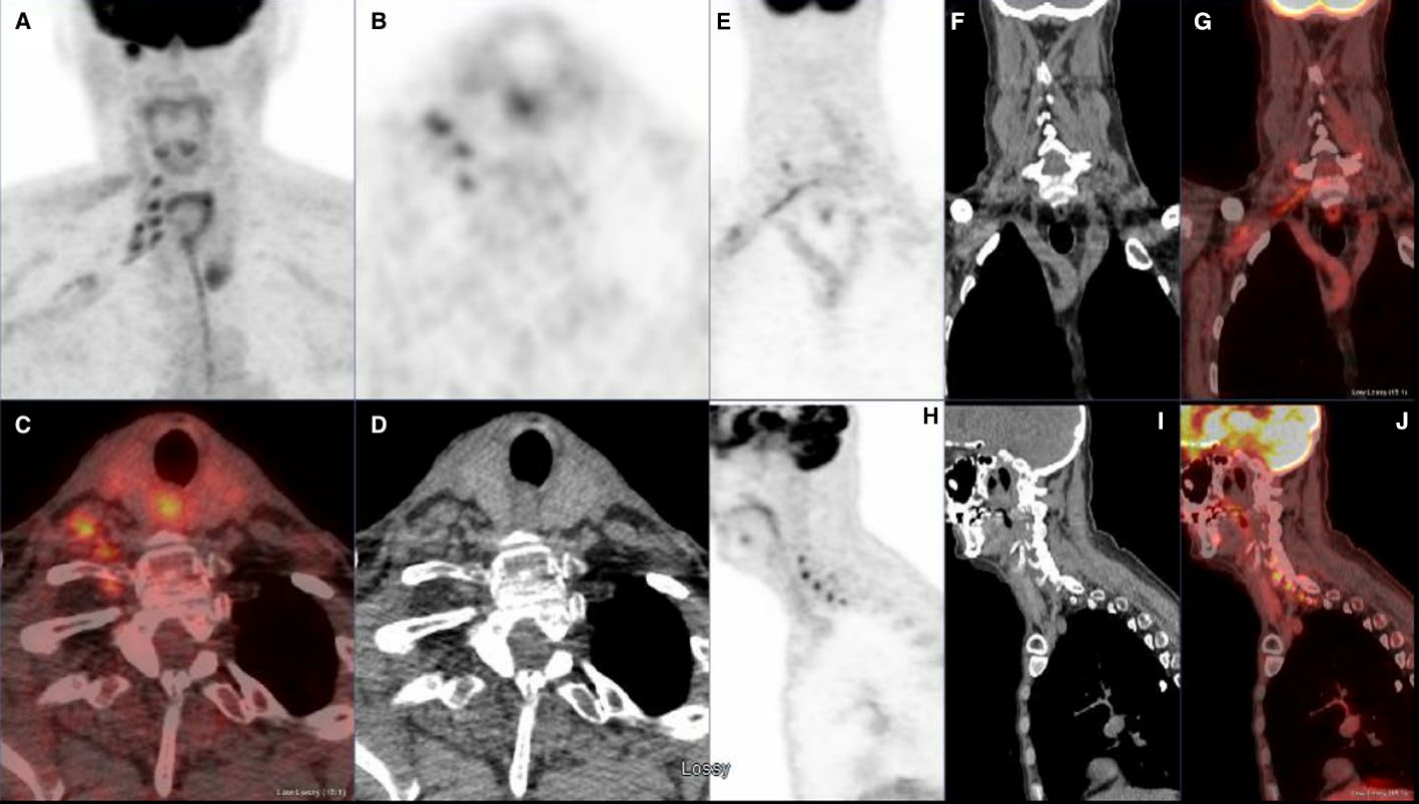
Axial PET, fused and CT images (B, C and D) at the level of exit foramina in lower neck. Note intense uptake by the nerve roots on the right from C5 to T1. Coronal PET, CT and fused images (E, F and G) and Sagittal PET, CT and fused images (H, I and J) confirm the uptake in the distribution of the brachial plexus
